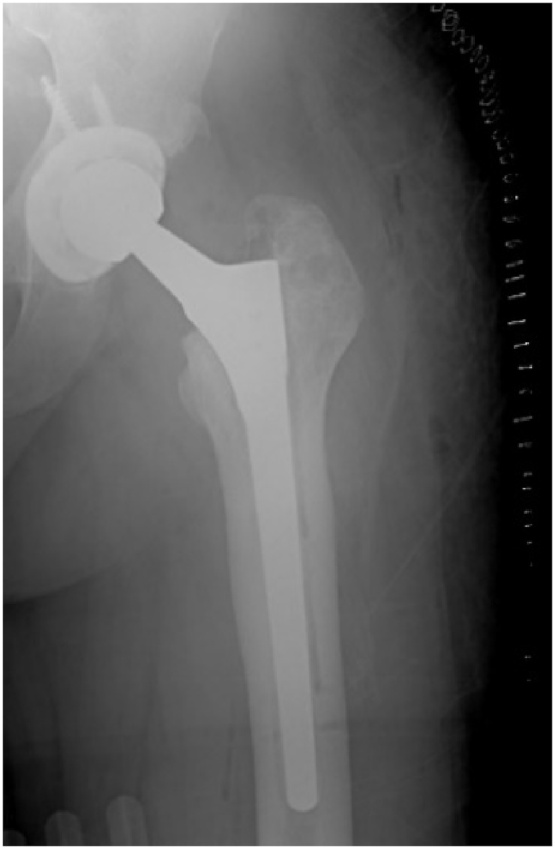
Immediate postoperative X-ray of the left hip arthroplasty performed using the Wagner SL (Zimmer Biomet, Warsaw, IN, USA) implant.
